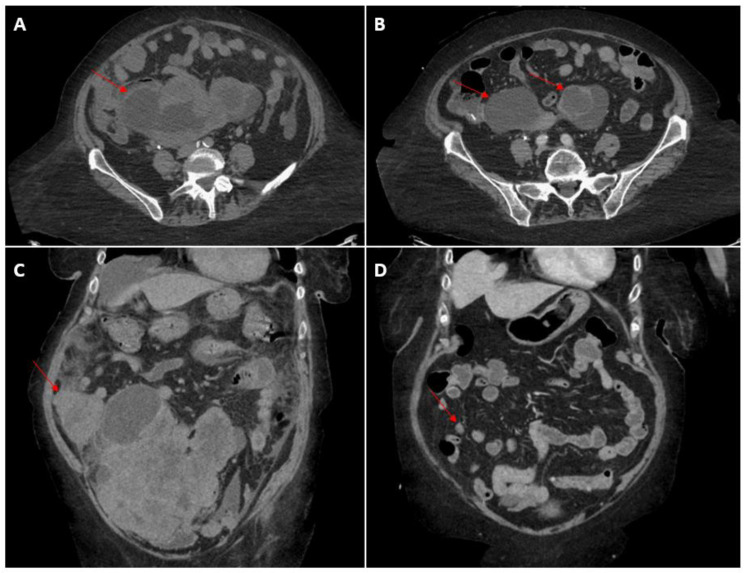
Representative computed tomography images after 4 cycles of chemotherapy. (A): pelvic mass on presentation. (B): pelvic mass after 4 cycles of carboplatin and paclitaxel. (C): right peritoneal implant on presentation. (D): right peritoneal implant after 4 cycles of carboplatin and paclitaxel.
